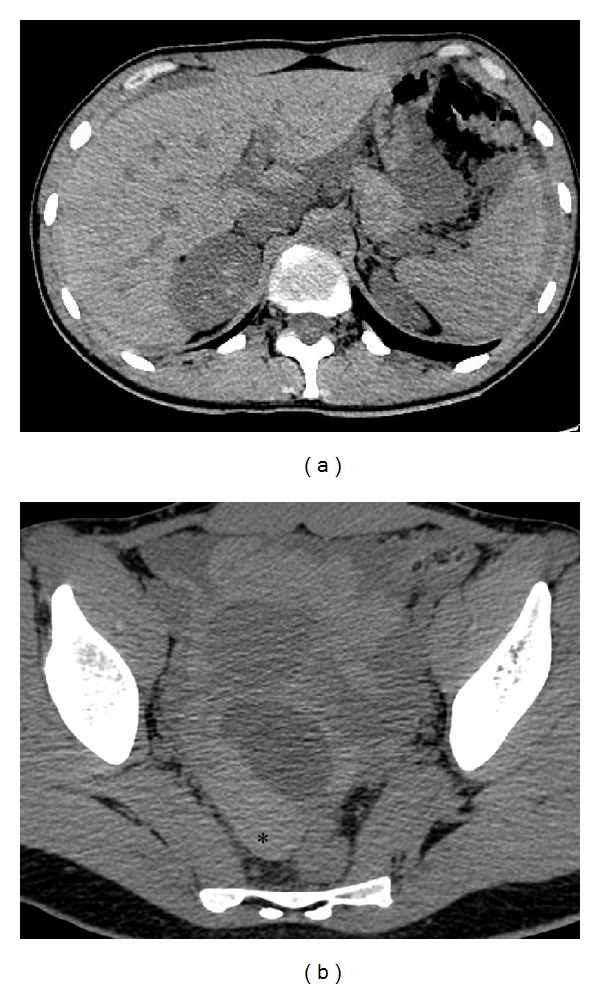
Unenhanced CT axial images (a, b) show diffuse peritoneal effusion in abdomen with different attenuation values that progressively increase from the upper abdomen (a) to the pelvis (b) where it becomes strongly hyperdense (60–65 HU,*) for the presence of blood component. (b) Low-density cyst is appreciable in the right adnexal area.
